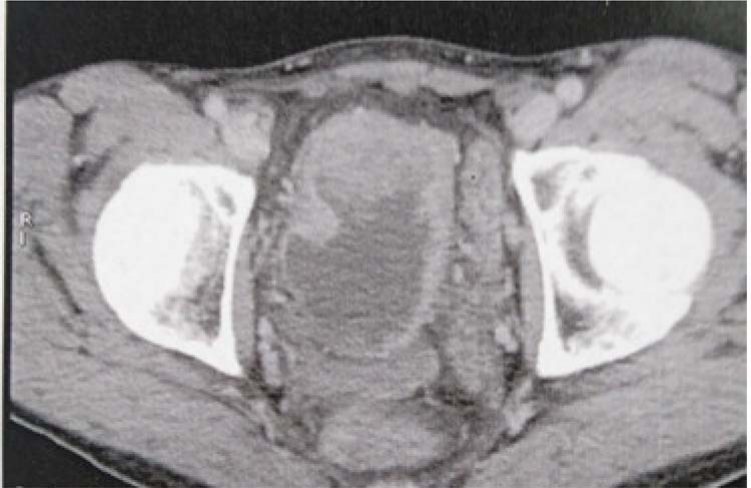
CT revealing a bladder tumor of 5 × 5 cm over the dome and anterior wall.
